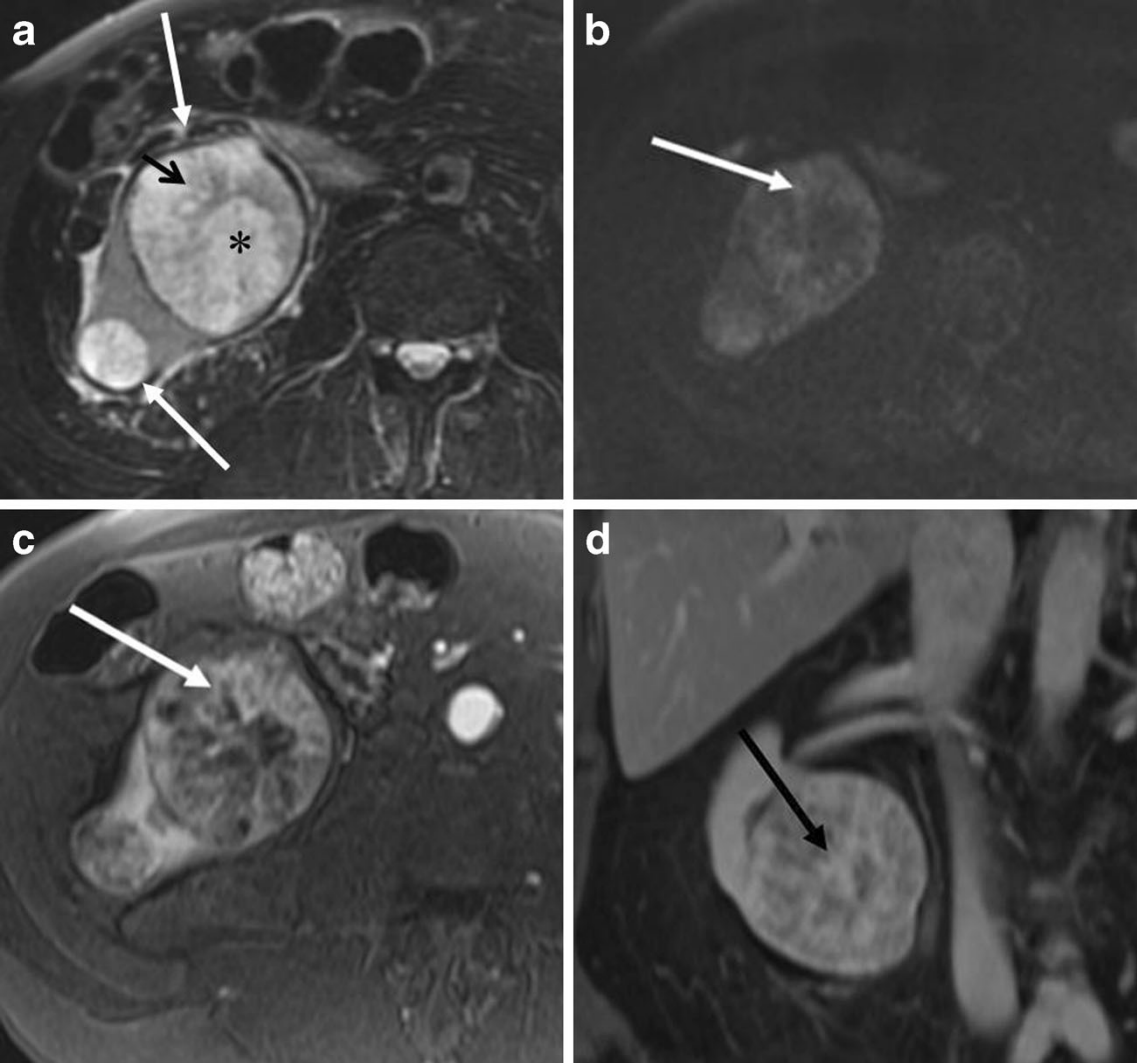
A 74-year-old woman with multifocal renal oncocytomas. a The axial T2-weighted FSE MR image with fat saturation shows two heterogeneous masses in the right kidney (*arrows*). The tumours consist of a mixture of intermediate (*small arrow*) and high signal (*asterisk*) components. b The axial diffusion weighted image (*b* factor = 800) shows the area of moderate signal on the T2-weighted image to be relatively high signal (*arrow*), suggesting increased cellularity. c The arterial phase of the axial T1-weighted GRE image with fat saturation demonstrates the area of intermediate signal on the T2-weighted image to be hypervascular (*arrow*). d Post-contrast coronal T1-weighted GRE image with fat saturation shows partial enhancement in the area of increased signal on the T2-weighted image (*arrow*)
